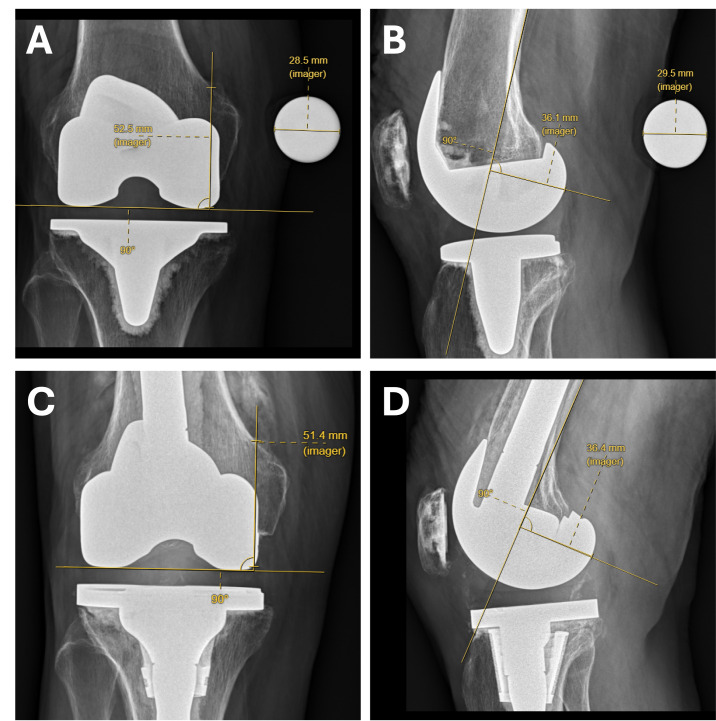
Anteroposterior (AP) and lateral radiographs of the total knee arthroplasty of a 64-year-old male at (a and b) prerevision, and (c and d) six weeks post-revision. (a and c) AP radiographs were used to calculate joint line position, while (b and d) lateral radiographs were used to measure posterior condylar offset at the corresponding timepoints.
